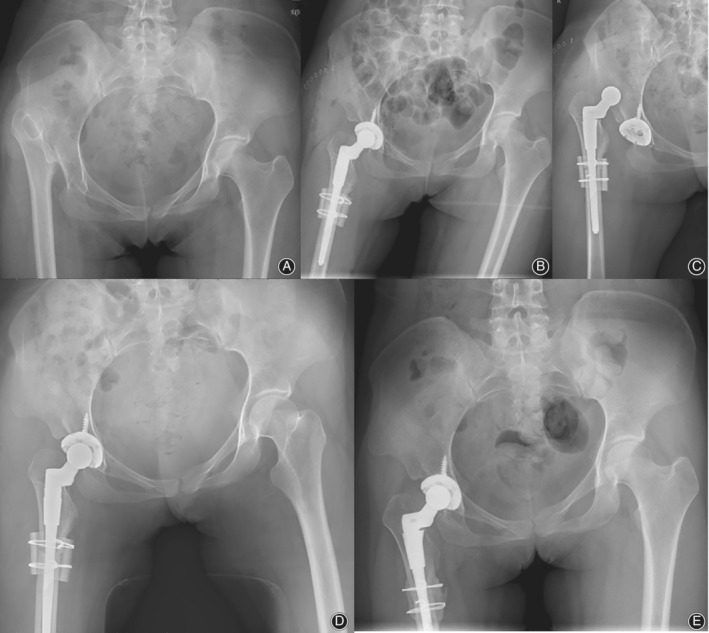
Radiographs of a 56‐year‐old woman with unilateral Crowe type IV DDH. (A) preoperative radiographic image showing high dislocation of the right hip without a false acetabulum. (B) Radiographic image at post‐operative day 1 showing that THA with a 22‐mm metal femoral head was performed in the right hip. (C) Dislocation occurred at post‐operative day 5. (D) Closed reduction was performed. (E) At the 4‐year follow‐up, no further dislocation or loosening of the component occurred.
